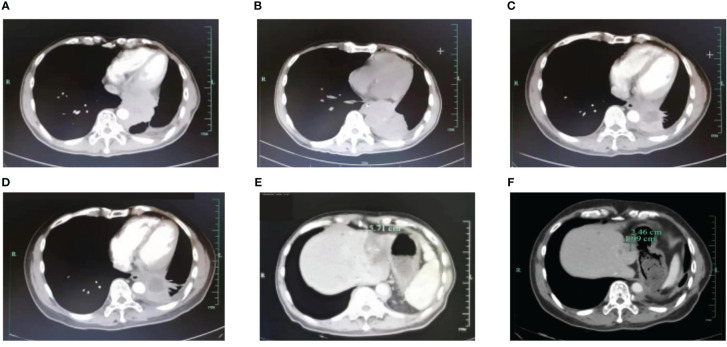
Radiographical changes. (A) PET/CT scan shows the failure of AP+Bevacizumab treatment in March 2019. (B) CT scan shows the failure of toripalimab+paclitaxel in July 2019. CT shows a durable response to alectinib in December 2019 (C) and 2020 (D). (E) PD on ensartinib in February 2022. (F) CT shows a partial response to lorlatinib in December 2022. AP, pemetrexed plus cisplatin; PD, progressive disease; PET/CT, positron emission tomography/computed tomography.
